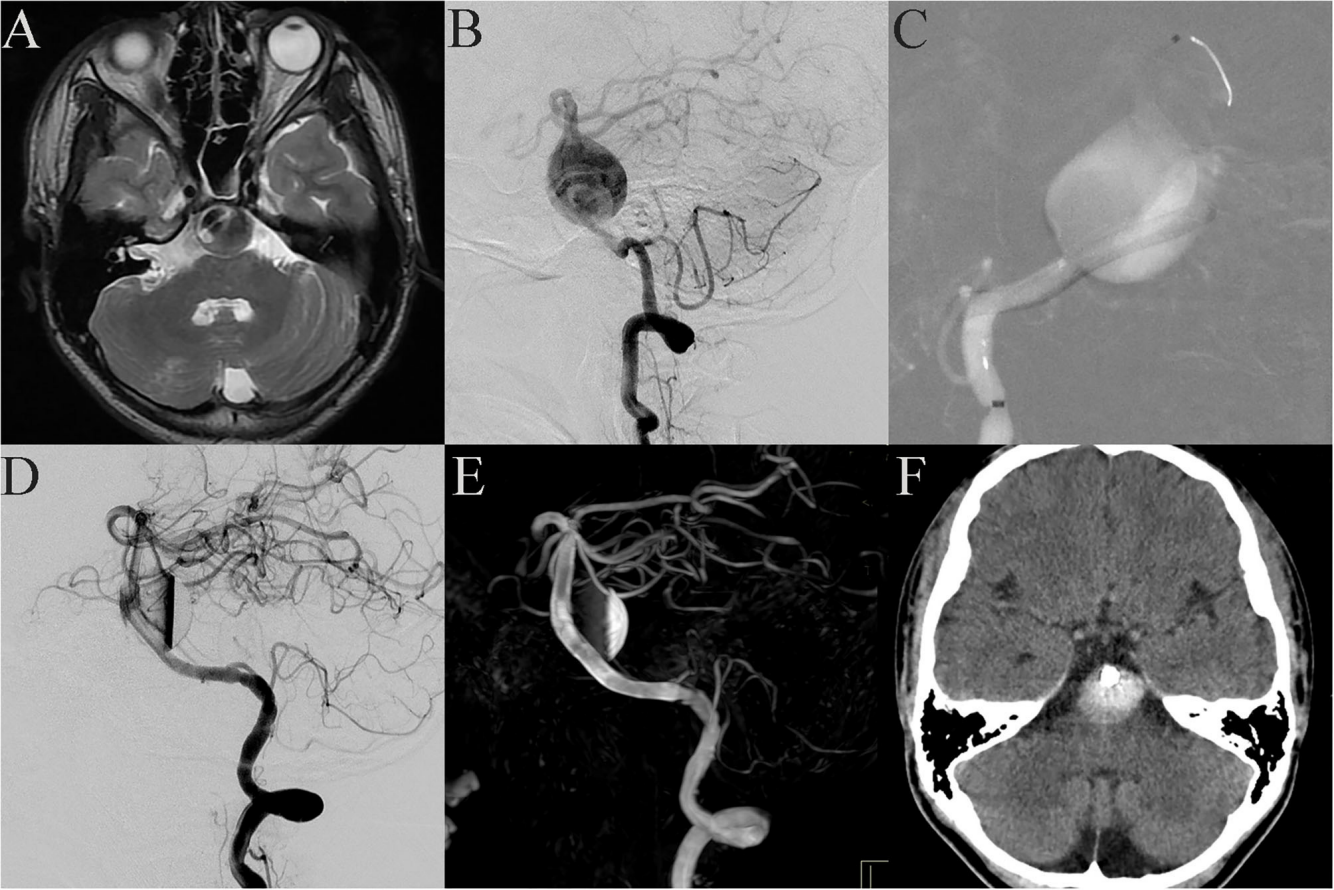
Case 18: Basilar trunk containing a giant fusiform aneurysm (A, MRI; B, lateral projection by DSA). During the stent bridging, the distal end of the stent easily fell into the aneurysm cavity (C). Post-embolization angiograms showed contrast stasis (D). Dyna CT showed good vascular reconstruction (E). One day post-operation, the patient gradually presented dyspnea and hemiplegia. Emergent cerebral CT showed no obvious bleeding (F).
